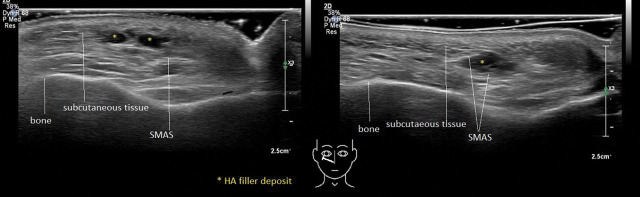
Ultrasound images of case number 2. Yellow asterix: hyaluronic acid (HA) deposits. In the left image, 2 HA deposits are correctly injected in the subcutaneous layer. In the right image (case number 2), the HA deposit is injected between layers of the SMAS, visible as a white lines covering the deposit both ventrally and dorsally. Note that the subcutaneous tissue above the deposit is pushed up and has become thinner. SMAS, superficial musculoaponeurotic system.
